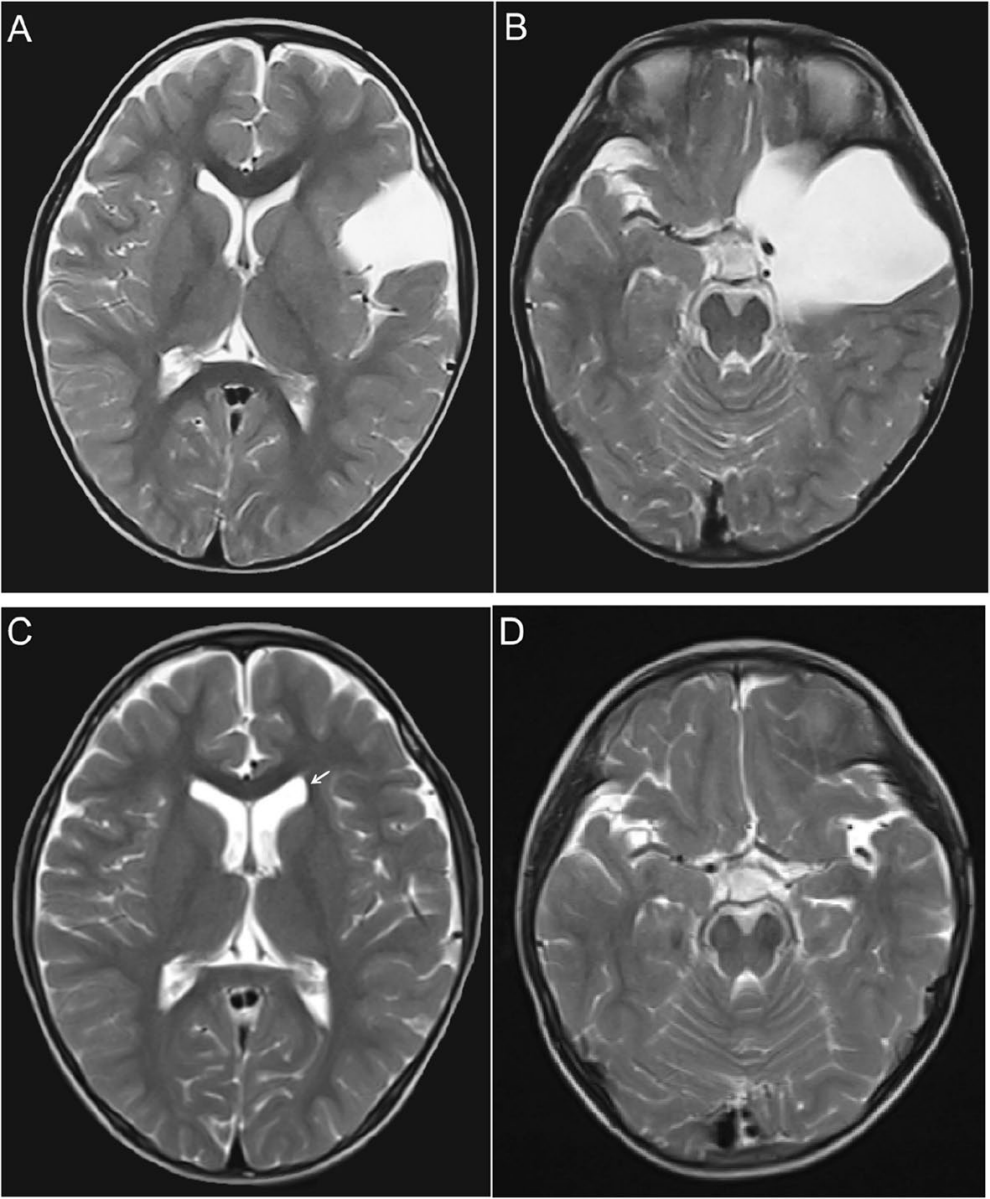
A 4-year-old boy presented with progression of AC size. A, B Preoperative axial T2-weighted Magnetic resonance image of the head showing Sylvian AC on the left and bilateral symmetrical ventricles.C, D The same slice of axial T2-weighted MR image showing the disappearance of the cyst and slightly dilated ipsilateral ventricle (arrow) on 12 months follow-up MR imaging
